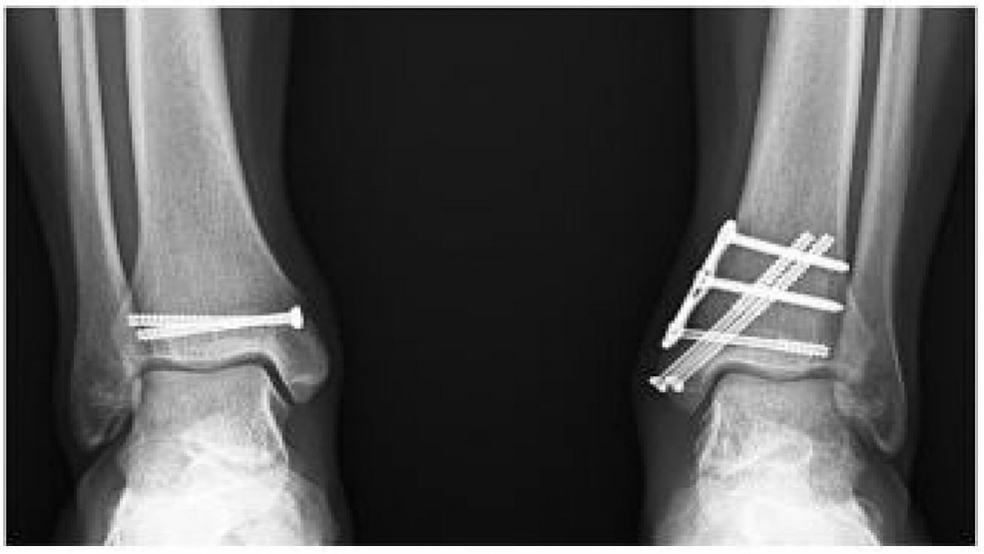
Three-month post-operative radiograph demonstrating routine healing of bilateral medial malleolus stress fractures.
